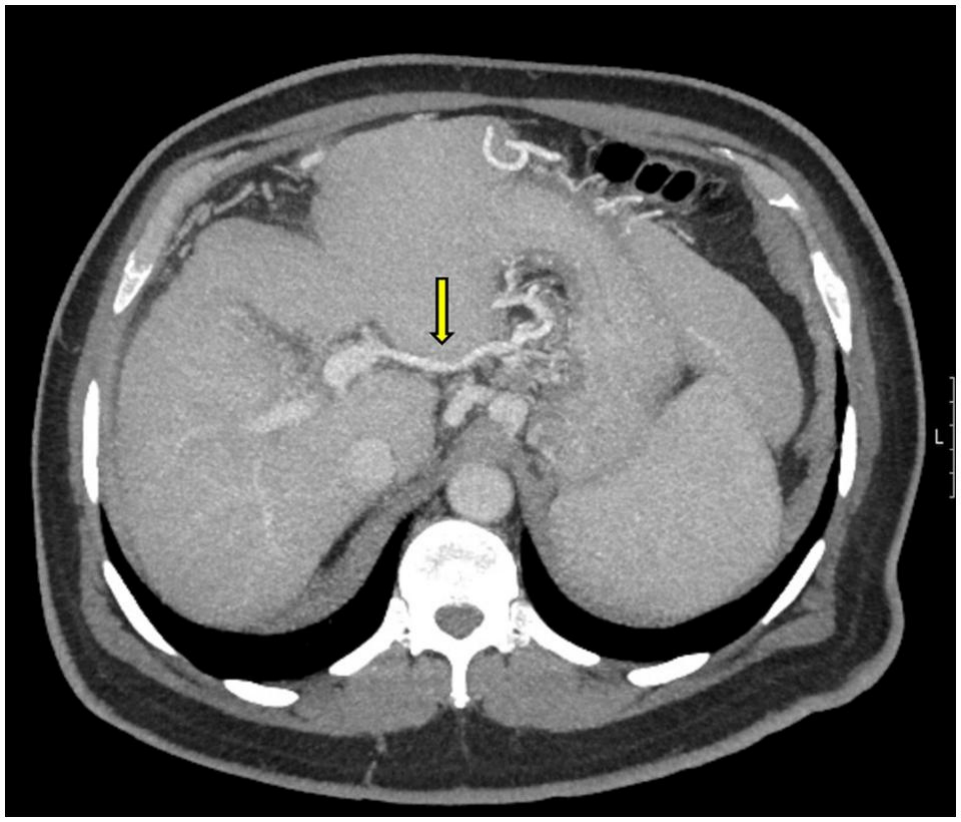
Axial portal phase contrast-enhanced CT MIP image shows the aberrant left gastric vein directly draining into the left portal vein (arrow), consistent with Type 3 aberrant left gastric vein.
